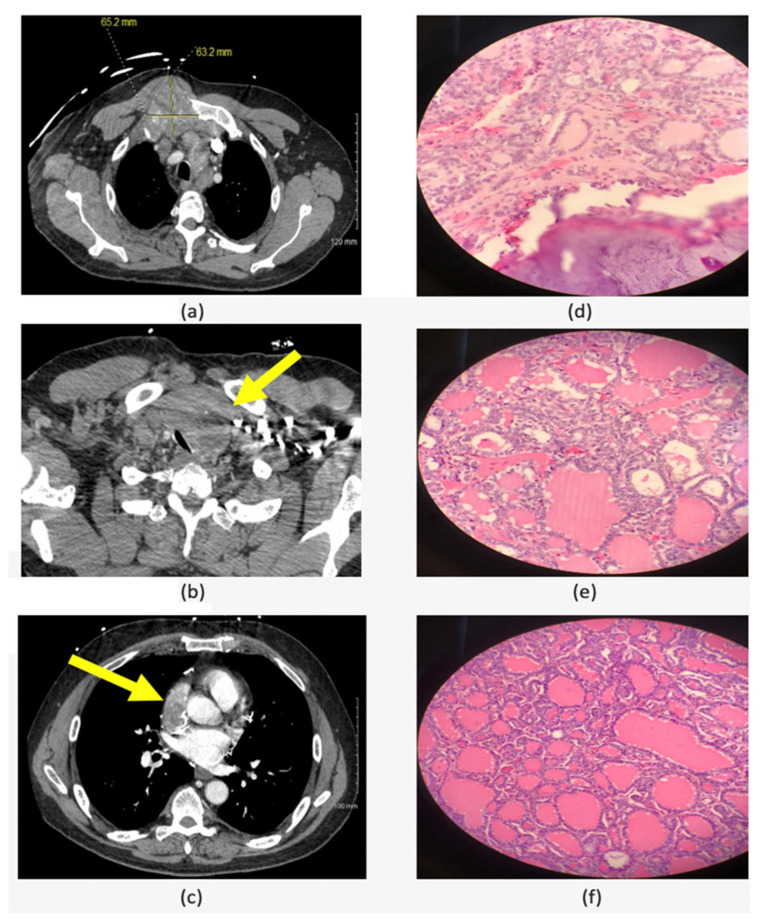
Left column is showing the neck and chest CT scan: (a) 6.5 cm right manubrial costal junction mass; (b) left thyroid lobe mass with tracheal compression (c) 5.1 cm superior vena cava (SVC)/right arterial mass. Right column is showing the final pathology from all the resected sites which showed follicular variant of papillary thyroid carcinoma: (d) chest wall metastasis; (e) left thyroid tumor; (f) right atrium metastasis.
